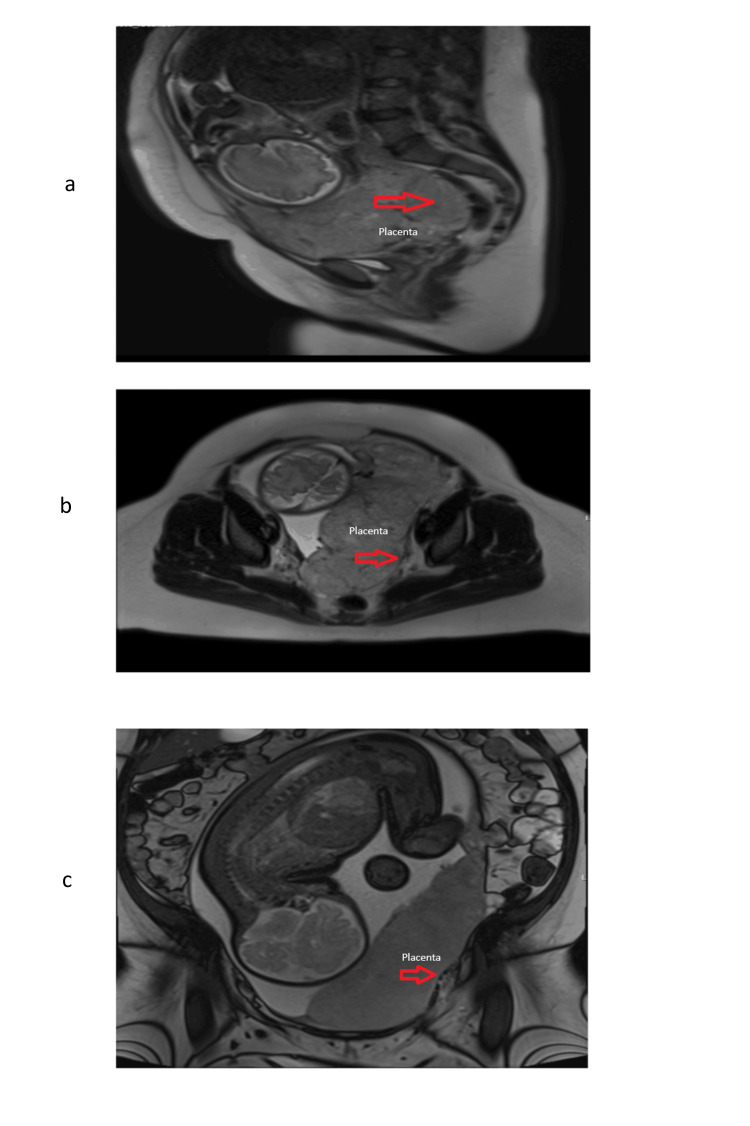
Placenta accreta. Figures (a-c) show MRI images of placenta accreta with a segment of percreta.
